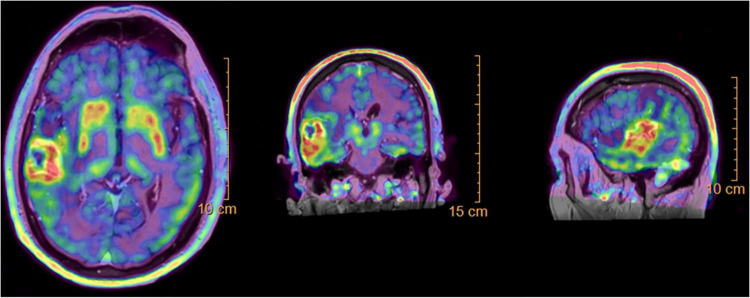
Brain ^18^F-fluorodopa positron emission tomography-computed tomography (FDOPA PET-CT) scan showing hypermetabolic activity in the right temporal lobe lesion, with a maximum standardized uptake value (SUVmax) of 3.38 g/ml, indicating tumor viability and progression.
